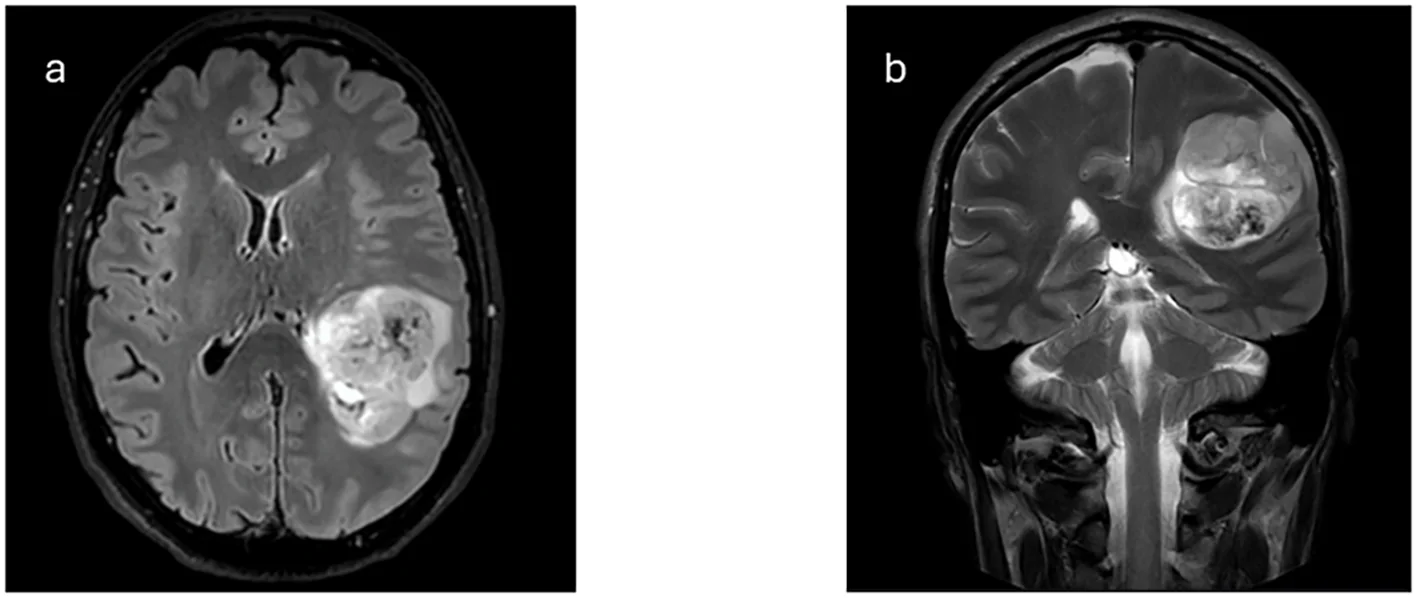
Preoperative brain MRI. (a) Axial FLAIR and (b) coronal T2 images showing a 60 × 45 × 45 mm left frontoparietal intra-axial mass with extensive cystic-necrotic components, surrounding vasogenic edema and associated mass effect.
